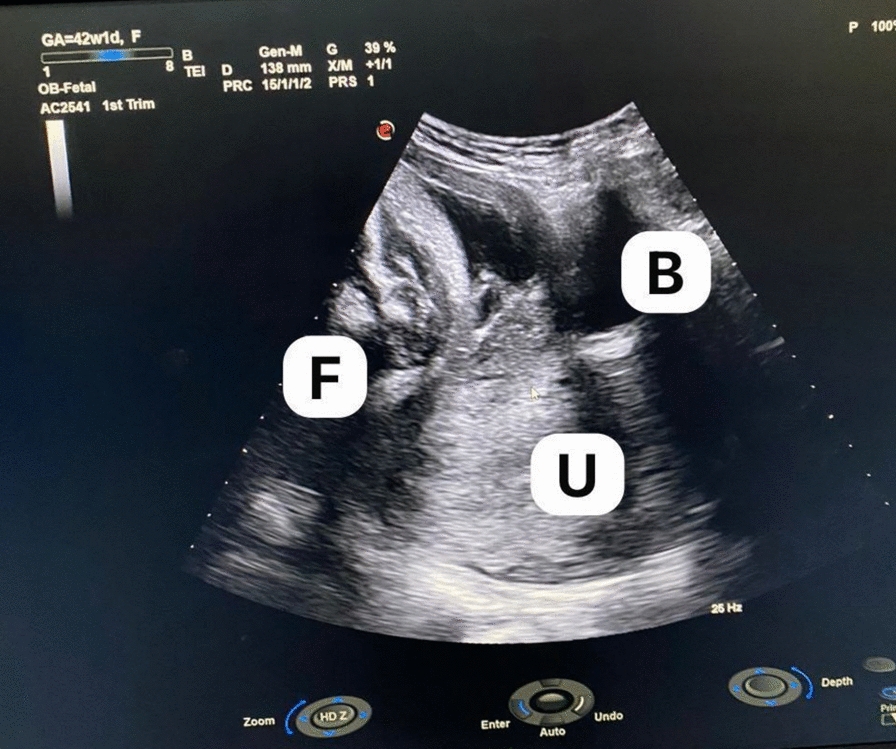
Ultrasound imaging from the initial presentation showing a breech fetus (F) that was initially misinterpreted as intrauterine. In retrospect, the fetus was located outside the uterine cavity (U). The maternal bladder (B) is also visualized
